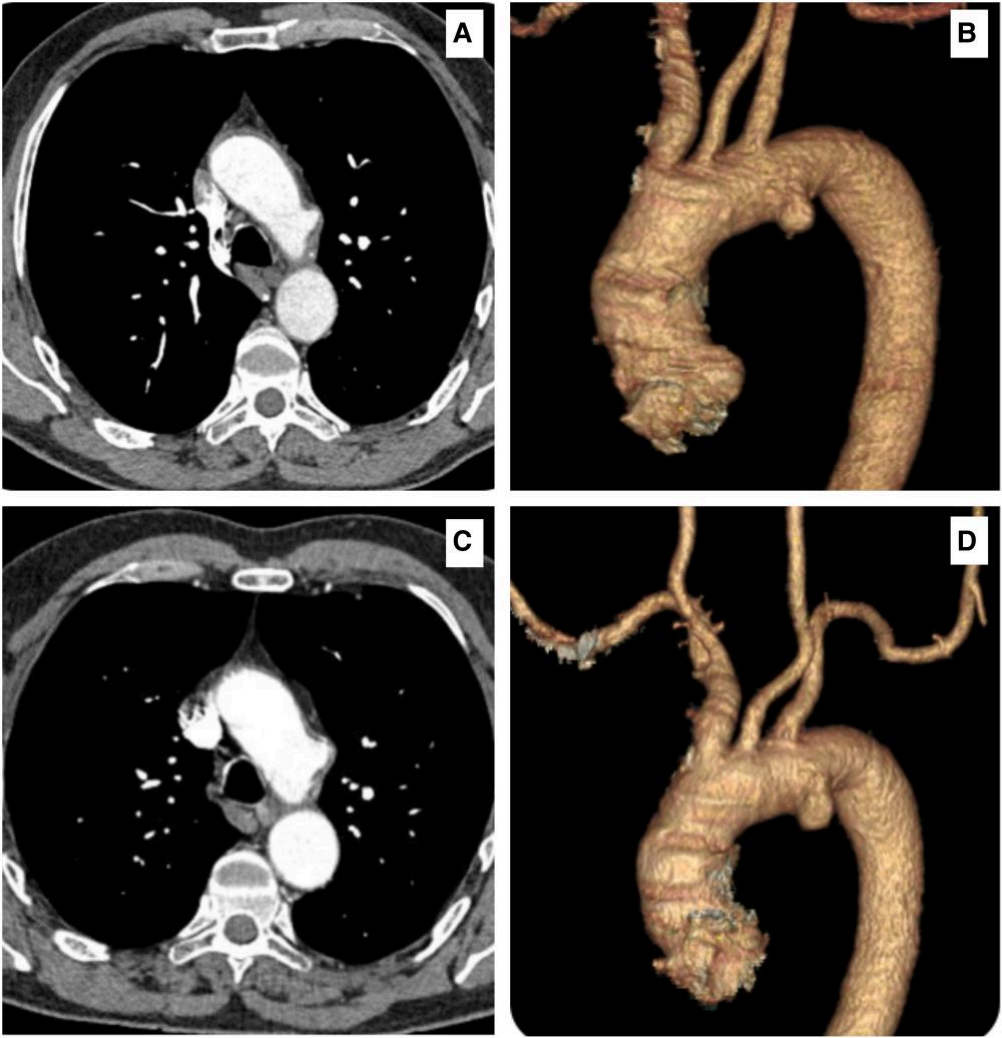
Computed tomography angiography scans and the 3D reconstructions, showing the following: (*A*, *B*) in July 2013, there was a 2.1 cm thoracic aortic arch diverticulum at the inner concavity of the aortic arch. (*C*, *D*) A 2.9 cm thoracic aortic arch diverticulum at the inner concavity of the aortic arch in February 2015.
